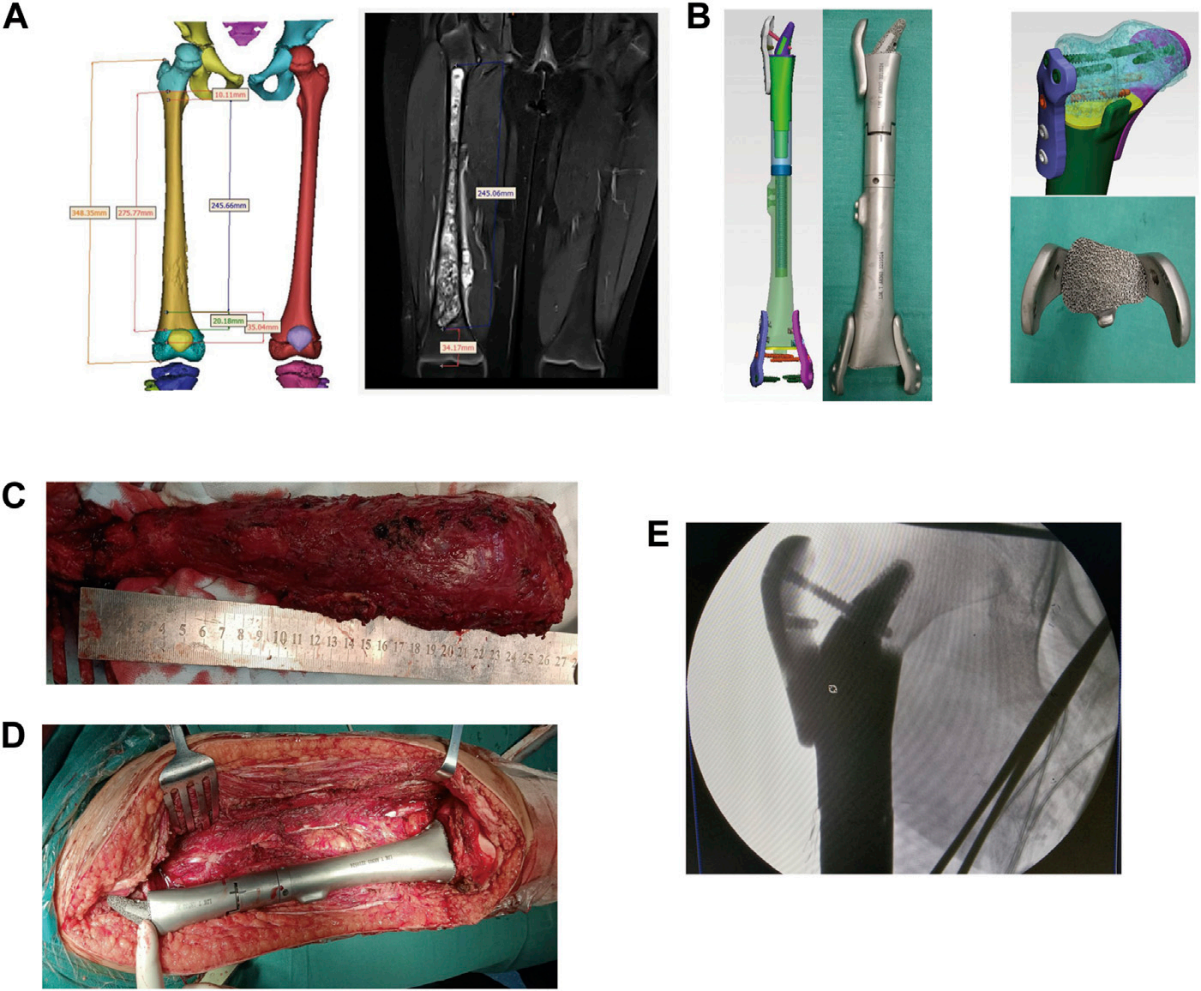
The reconstruction of the right tibia with a 3D-printed scaffold of a patient with osteosarcoma. (A) The design of the 3D-printed scaffold based on the MRI results. (B) The custom 3D-printed prosthesis components. (C,D): The length measuring of the osteotomy. (E) The fixing of the custom 3D-printed prosthesis on the bone defect.
